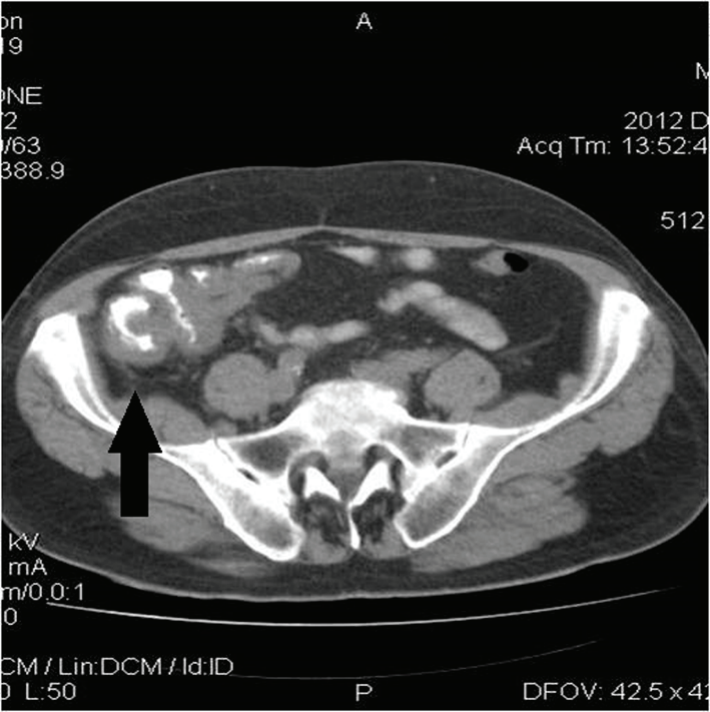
Enhanced abdominal CT demonstrates wall thickening of the cecum, the distal portion of the terminal ileum, and the lower portion of the ascending colon accompanied with pericolic inflammation, as indicated by the arrow.
